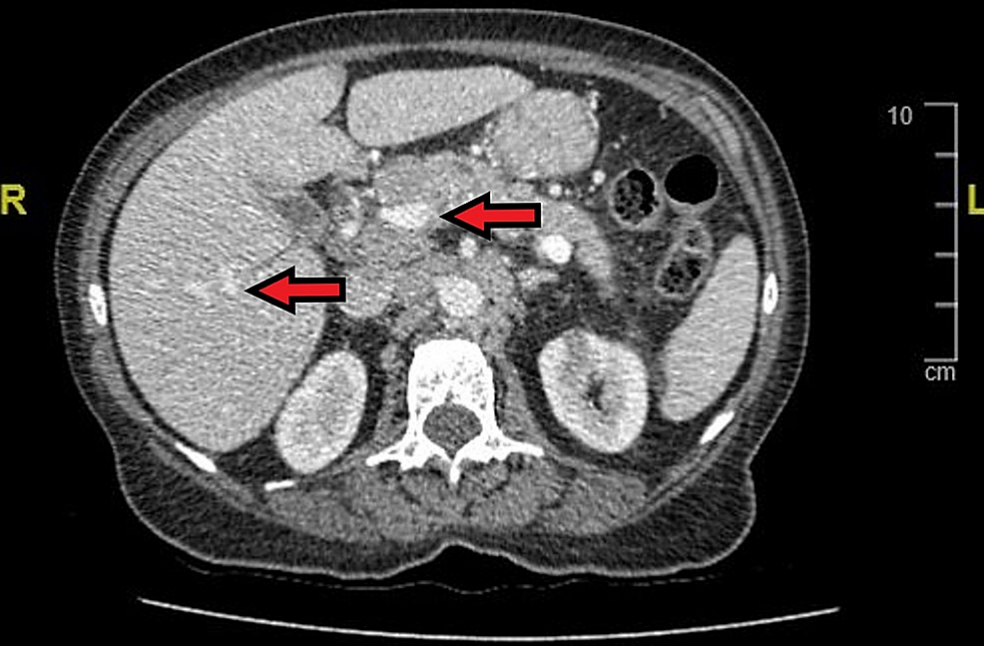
Transverse plane view of patient’s CT abdomen showing gastrinoma mass (arrows) in the pancreatic head with the appearance of metastatic lesions in the liver
